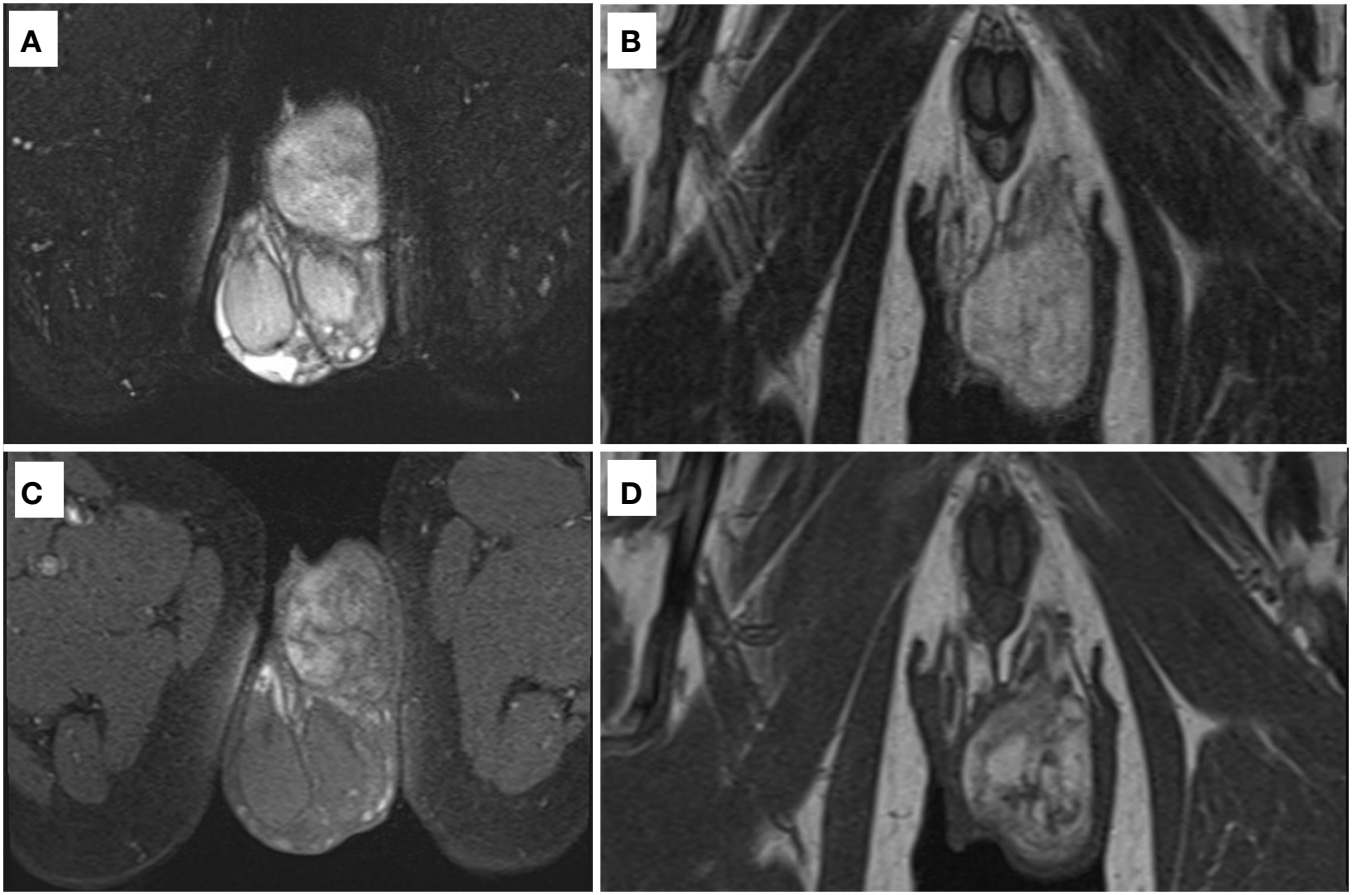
MRI. (A) T2-weighted image (axial view) with fat suppression. (B) T2-weighted image (coronal view). (C) T1-weighted image (axial view) with fat suppression. (D) T1-weighted image (coronal view).
